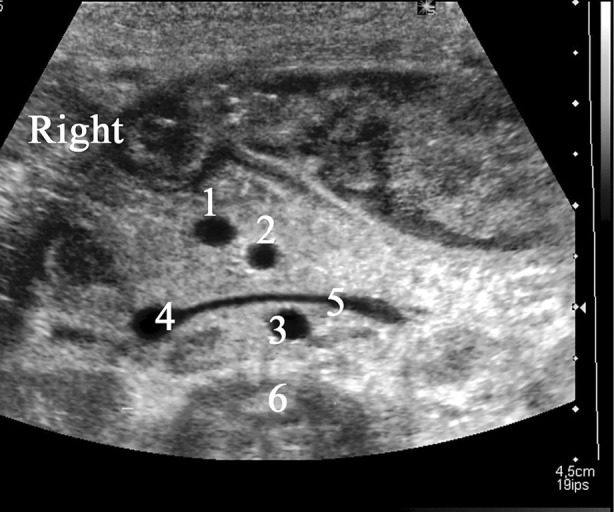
Pediatric abdominal ultrasound showing the gold standard section to analyze superior mesenteric vessels location. The superior mesenteric vein (SMV) (1) is normally on the right side of the superior mesenteric artery (SMA) (2). The two mesenteric vessels are visualized in a strict cross‐sectional plane. Between the aorta (3) and the SMA (2) the left renal vein (5) draining into the inferior vena cava (4) is visualized. Spine (6).
